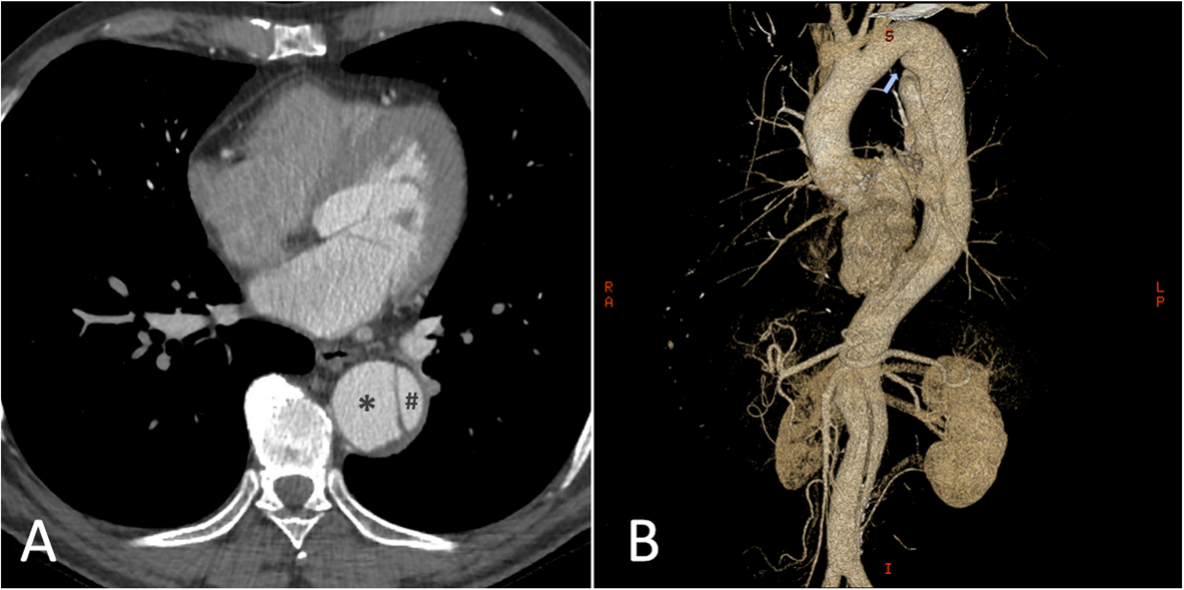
CTA image obtained 6 months after the acute event showing no progression of the aortic dissection. a Axial view showing the false lumen (*asterisk*) still prevailing over the true lumen (*hash*). b CTA 3D-scan showing the stability of the TBAD; the light blue arrow indicates the origin of the dissection
